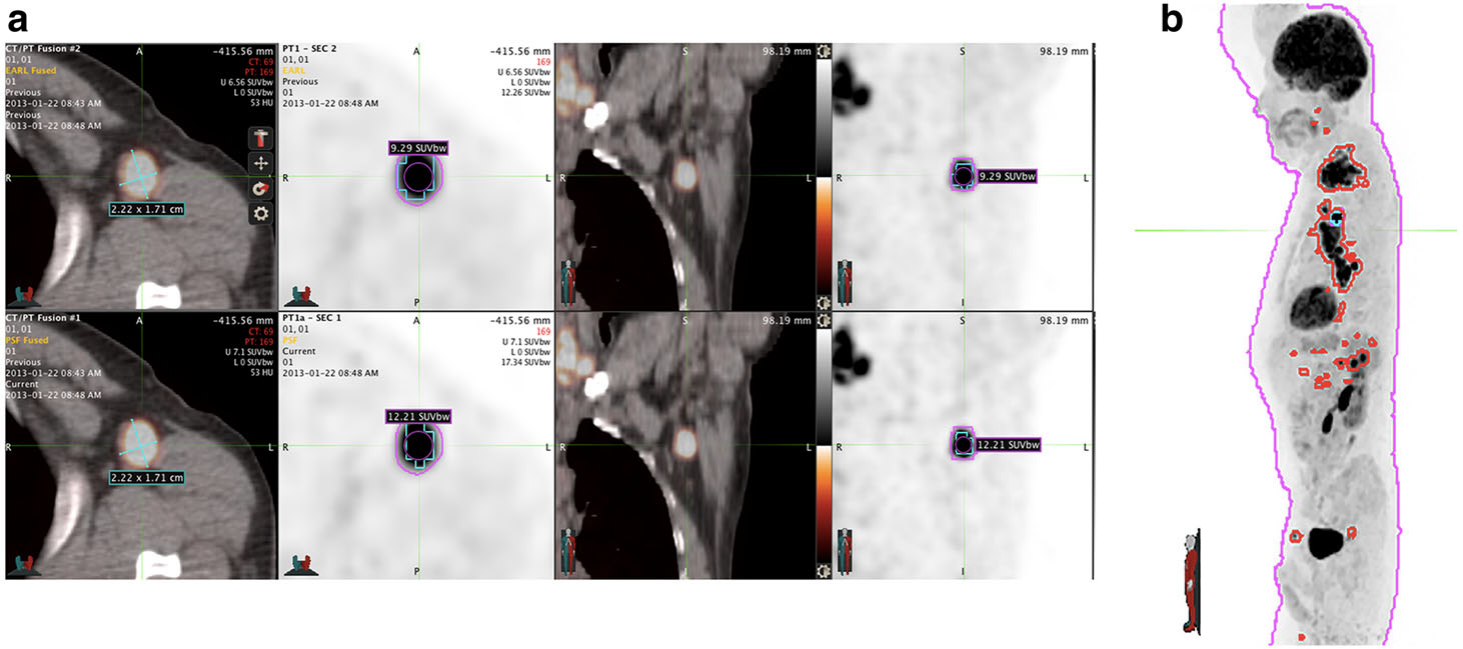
a Single lesion volume of interests (1) for entire lesion with (2) RECIST measurement and (3) 40% SUV threshold. b Whole body tumor burden maximum intensity projection-segmented based on the AC-CT, contour was then copied to the two PETAC reconstructions and sub-segmented to include only SUV values above 2.5 and normal tissue uptake was subtracted (i.e., brain and kidney)
